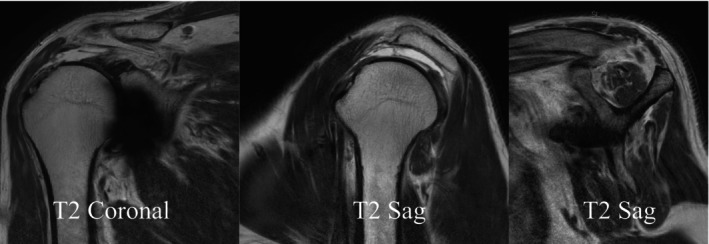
Preoperative MRI showing a large to massive rotator cuff tear involving the supraspinatus and infraspinatus with mild muscle atrophy, indicating an irreparable cuff tear.
